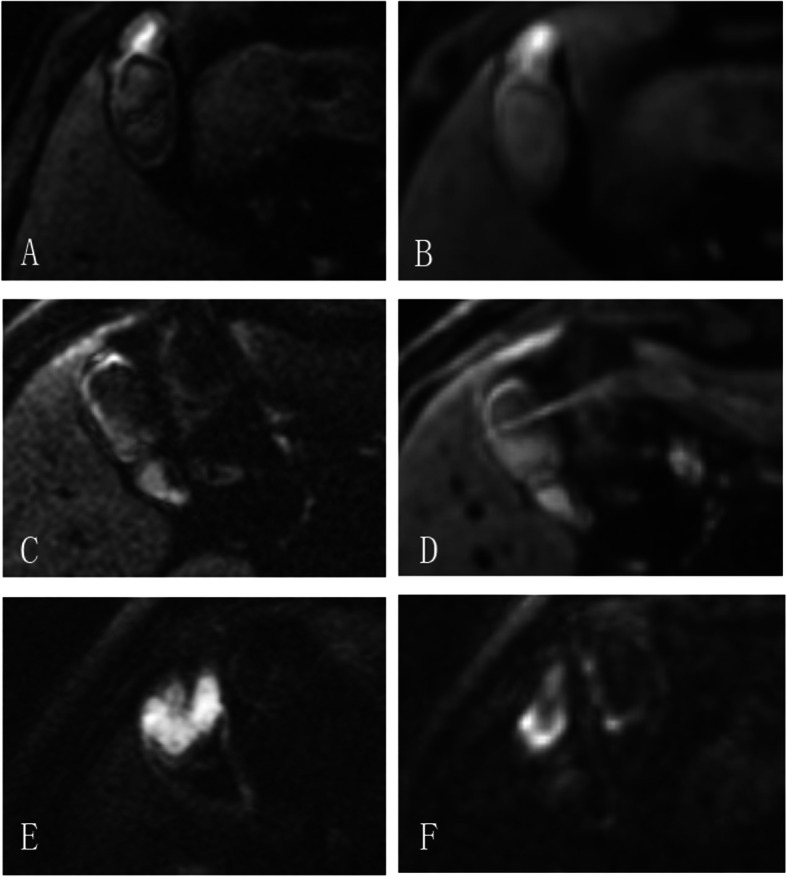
Images for subjective quality assessment. a, b: Assessment of sharpness and lesion conspicuity (gallbladder adenomyomatosis). a: r-FOV DWI image shows the soft tissue signal at the bottom of the gallbladder with a sharp margin, and the lesion features are clearly demonstrated. Subjective image quality scores of sharpness and lesion conspicuity are both 3. b: f-FOV DWI image shows the soft tissue signal at the bottom of the gallbladder with blurred edges and lower contrast comparing with Fig. A. The subjective image quality scores of sharpness and lesion conspicuity are both 2. c, d (gallbladder adenoma): Assessment of artifacts. c: r-FOV DWI image shows mild artifacts around the wall of the gallbladder. Subjective image quality score of artifacts is 4. d, f-FOV DWI image shows obvious artifacts. Subjective image quality score of artifacts is 3. e, f (gallbladder adenocarcinoma): Assessment of distortion. e: r-FOV DWI image shows a diffusion-restricted lesion at the bottom of the gallbladder without distortion. Subjective image quality score of distortion is 4. f: f-FOV DWI image shows the edge of the lesion with obvious distortion. Subjective image quality score of distortion is 2
